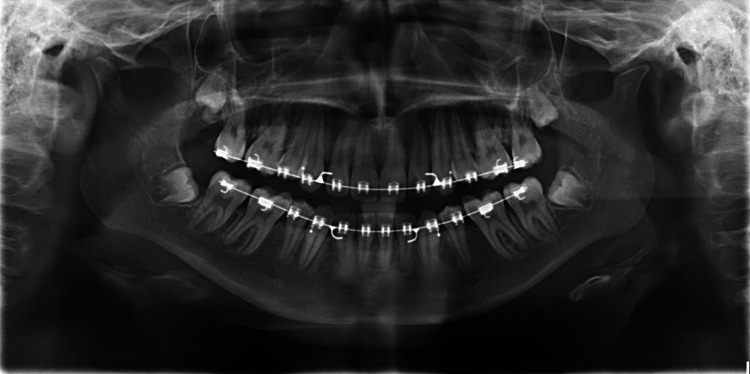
Pre-debond dental panoramic radiograph
